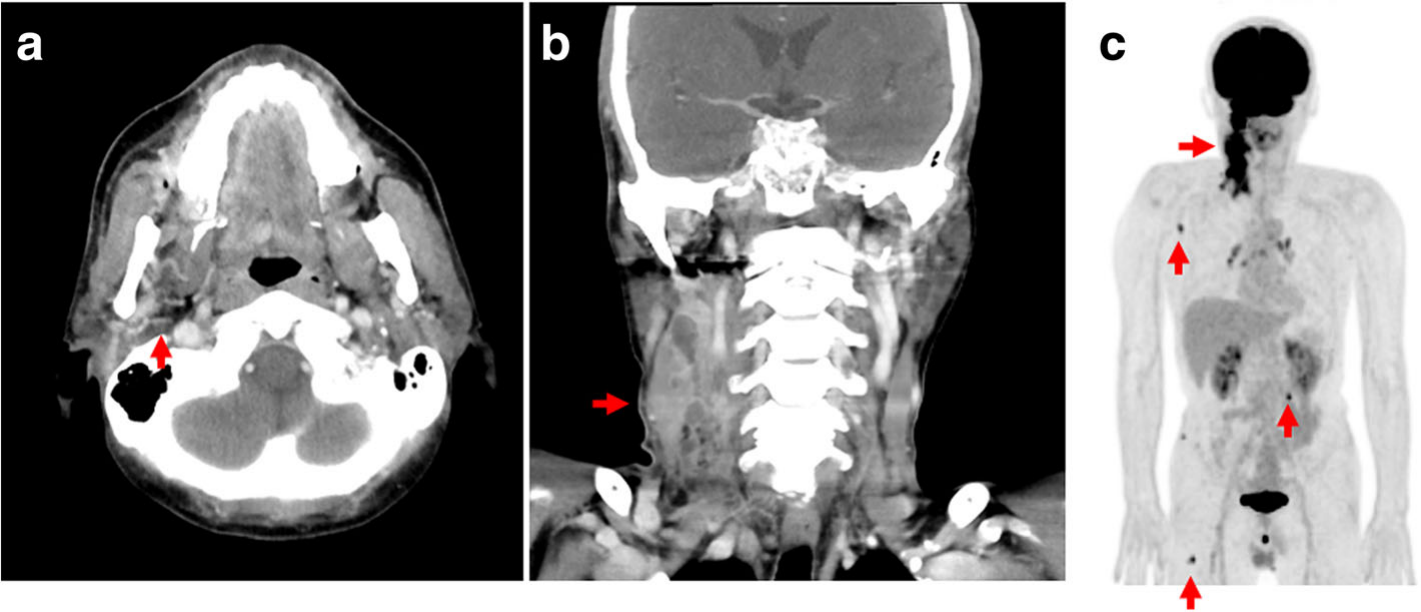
Patient computed tomography scan and positron emission tomography/computed tomography images. a and (b) Computed tomography showed a mass in the right buccal mucosa (*red arrow*) that extended superiorly to destruct the lateral wall of the maxillary sinus, inferiorly to the retromolar trigone, and laterally to the buccinator muscle and the anterior border of the masseter muscles, with multiple cervical lymph node enlargement. c Whole-body ^18^F-fludeoxyglucose positron emission tomography/computed tomography showed increased uptake in multiple lymph nodes in the right cervical area, right scapula and erector spinae muscles, and right femur (*red arrows*)
